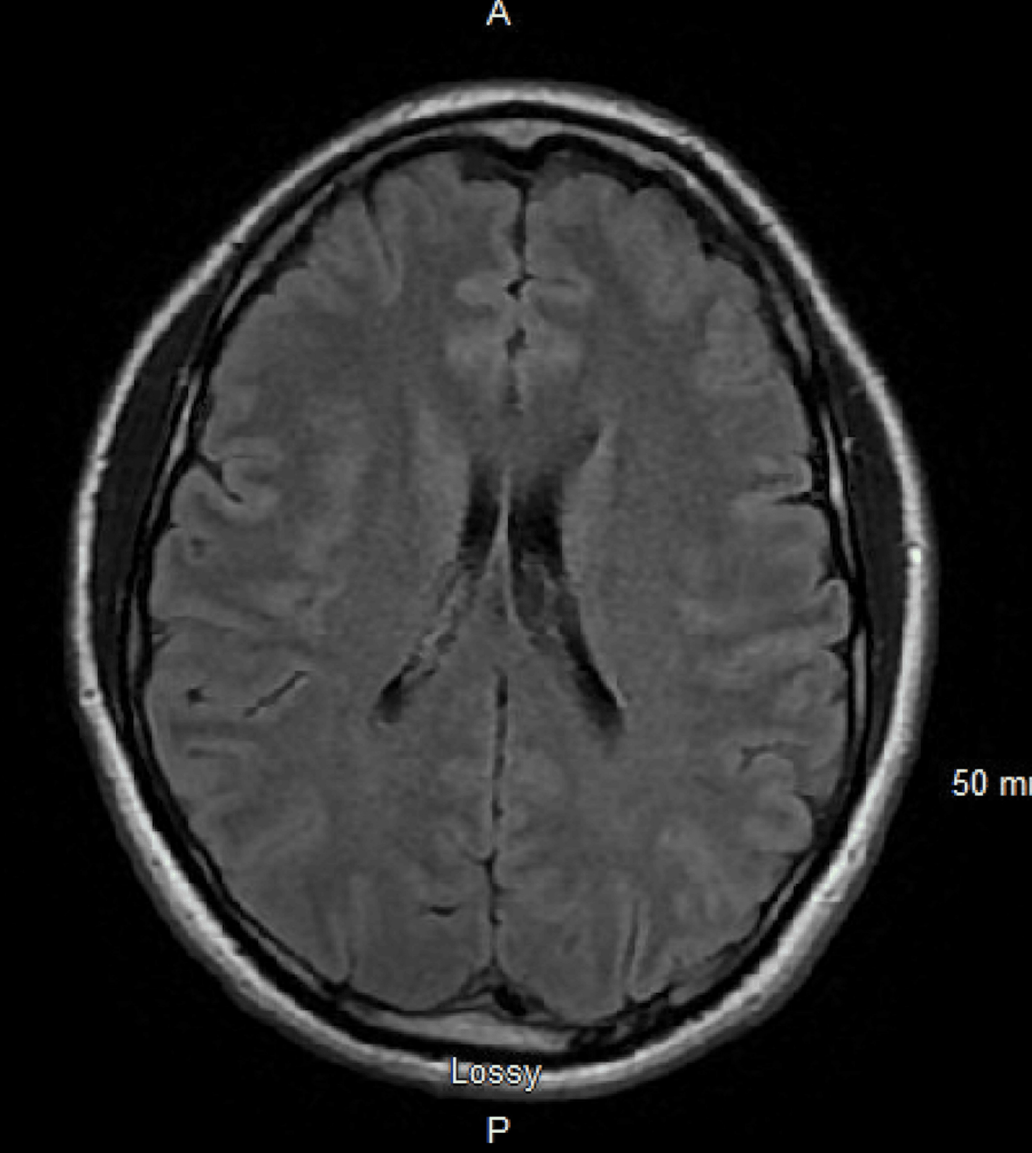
Head MRI showing no abnormalities
